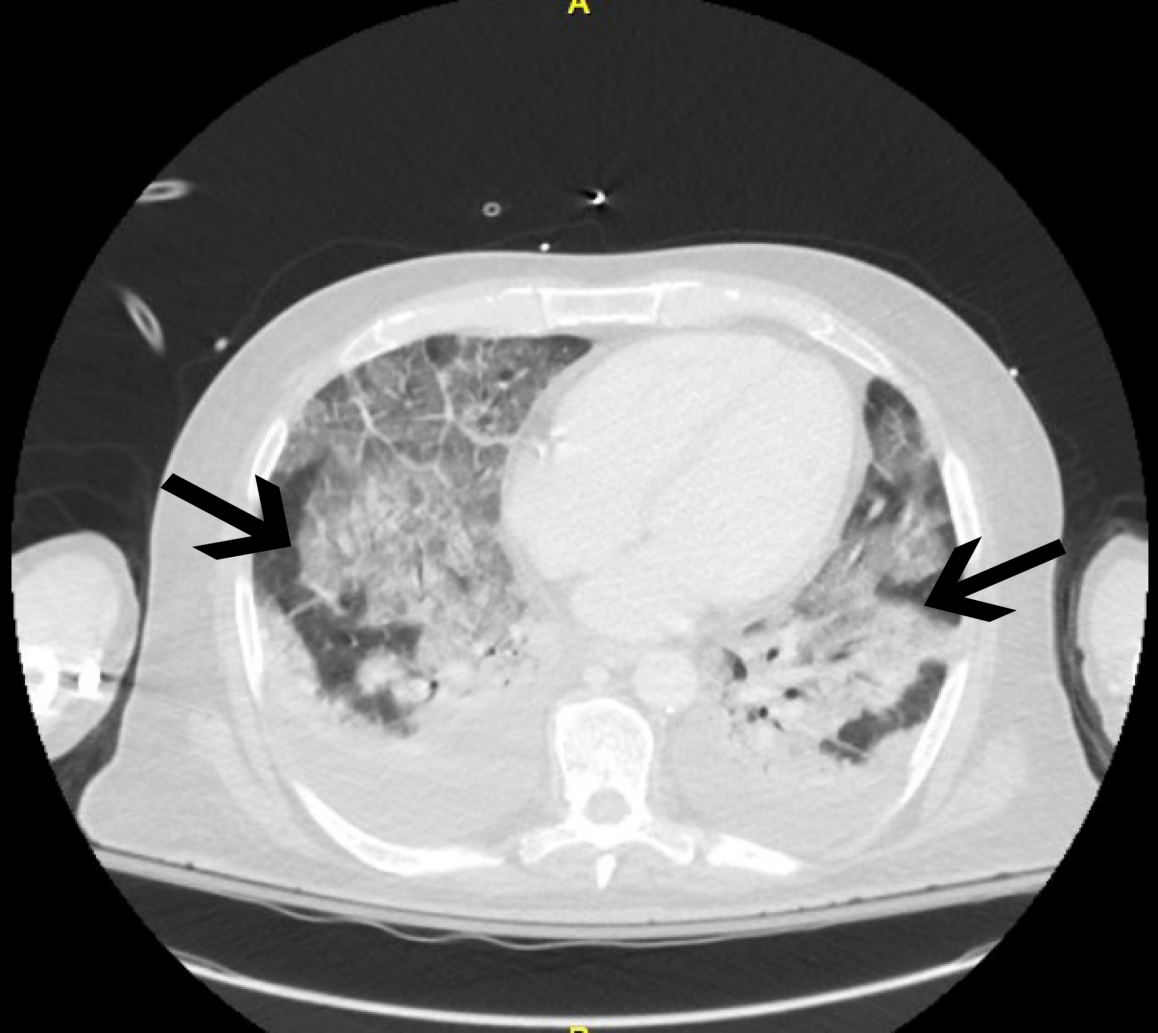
CT of chest with contrast demonstrates ground glass infiltrate and increased reticular markings (black solid arrows) in the dependent portion of both lungs suspicious for interstitial lung disease and possible early fibrosis
